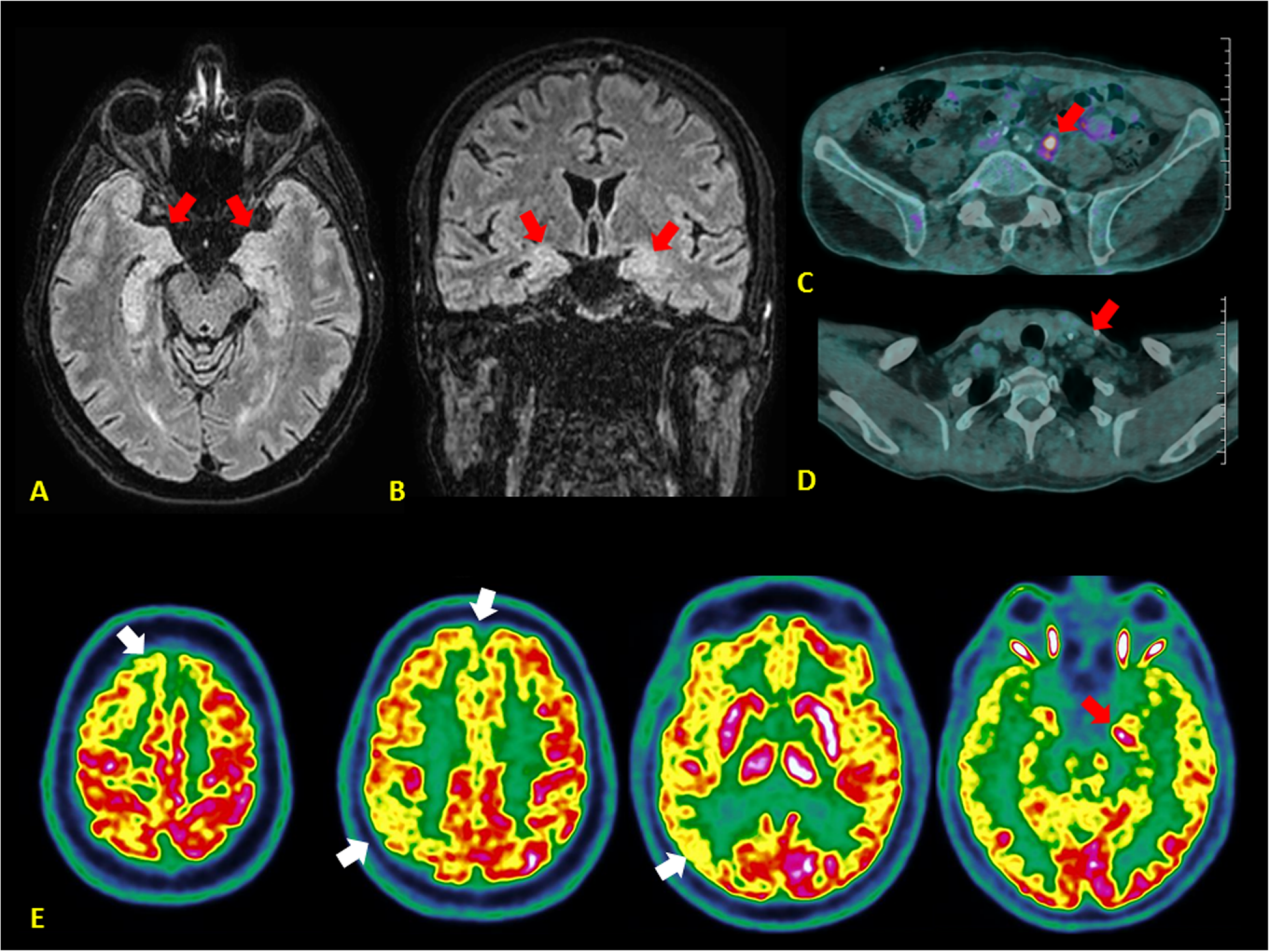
Tumor response to chemotherapy and radiological improvement of the PLE. a-b Reduction of the T2-weighted FLAIR signal of the para hippocampic gyrus and the hippocamps after 3 cycles of chemotherapy (brain MRI: coronal and axial section; red arrows). c-d Partial regression of the left, hypermetabolic peri-aortic adenopathy and complete response of the left, hypermetabolic supraclavicular lymph node after 6 cycles of chemotherapy (PET-scan; red arrows). e Residual, left hypermetabolism of the hippocampus (brain PET-scan; red arrow) and right prefrontal, parietal and occipital cortical hypometabolism (brain PET-scan; white arrows) after 6 cycles of chemotherapy
